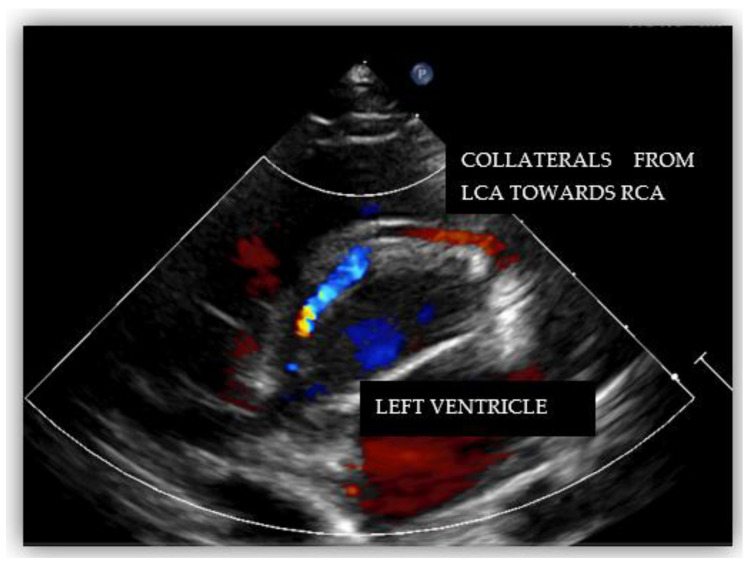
Echocardiography in parasternal short-axis showing collateral circulation.
